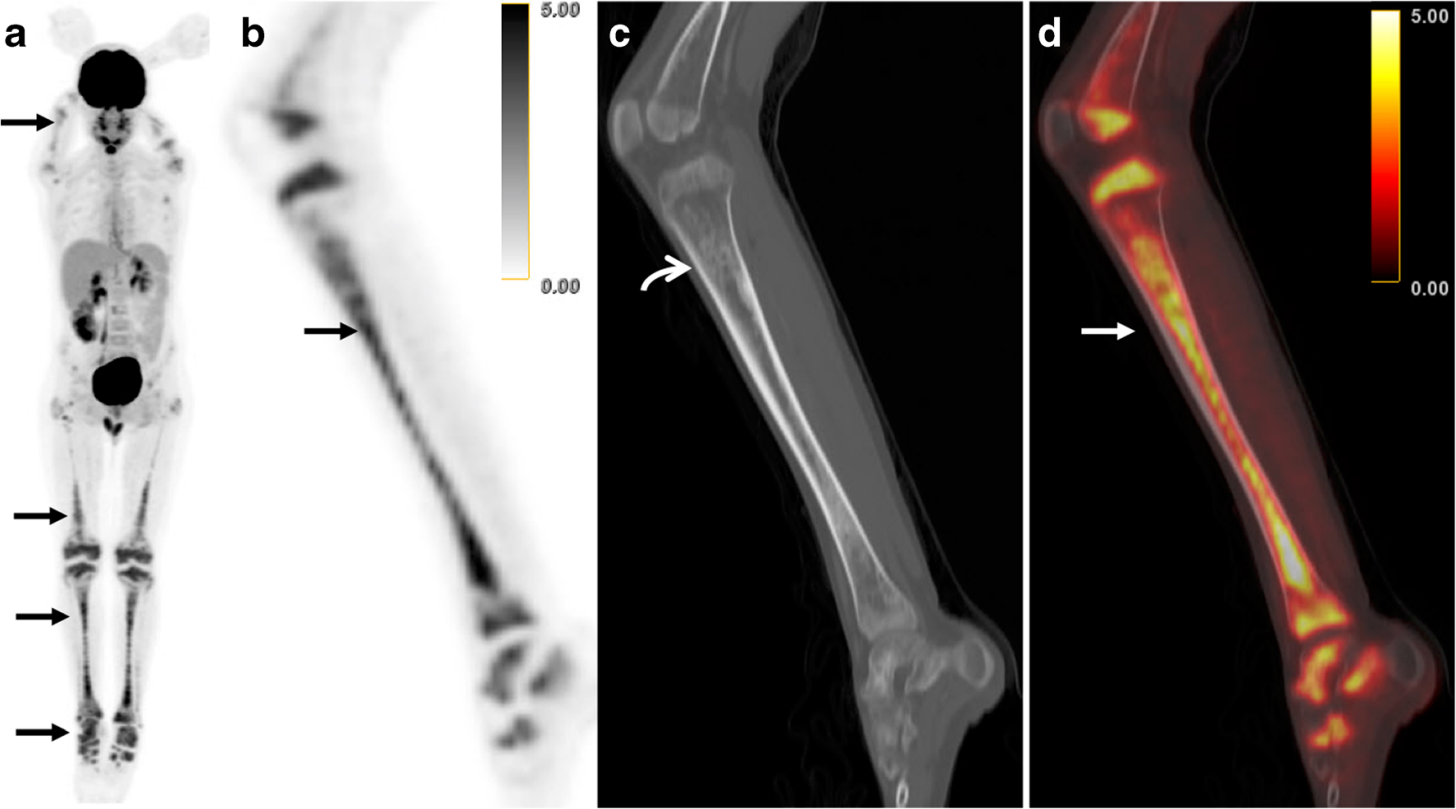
ECD detectable by both FDG PET and CT, but measurable only by mPERCIST, in an 18-year-old male. a FDG MIP demonstrates multi-focal abnormal FDG-avidity, greatest in the lower extremities (arrow). (b) Sagittal FDG PET, (c) sagittal CT, and (d) sagittal-fused FDG PET/CT centered on the left tibia demonstrate FDG-avid osseous ECD with an SUV_max_ of 5.2 (arrows). The CT demonstrates sclerotic correlates (curved arrow) that are detectable on CT, but not measurable by RECIST
